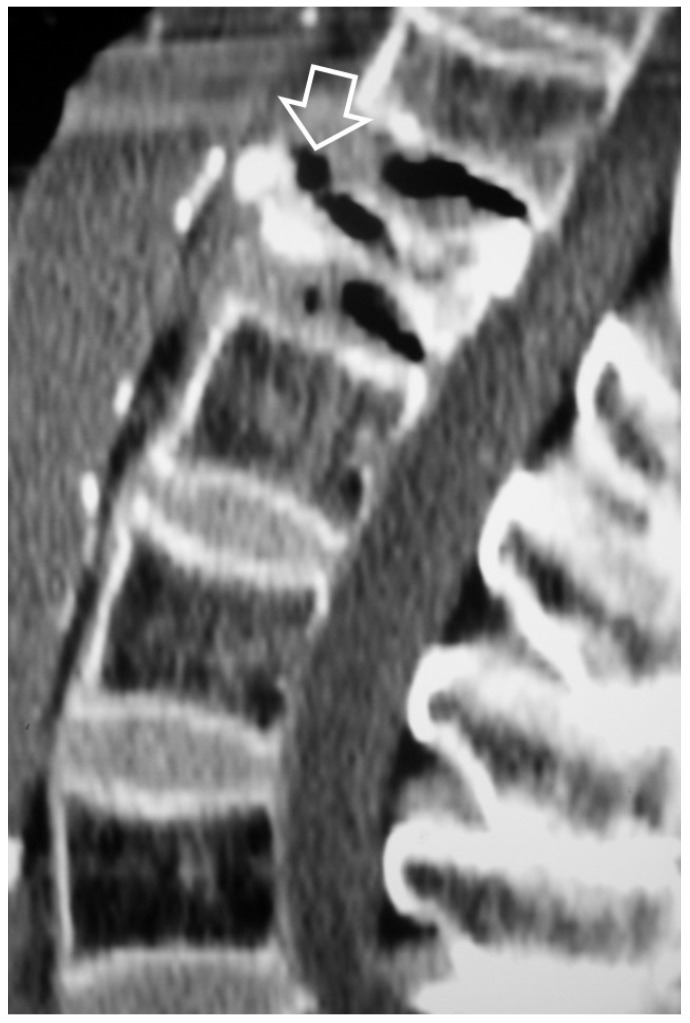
A 71-year-old female patient with an old osteoporotic fracture of the L1 vertebral body. CT sagittal reconstruction showing fragmentation and osteosclerosis, which may mimic tuberculous infection. The presence of gas within the osteonecrotic cavity under the endplate (arrow) suggests the correct diagnosis.
